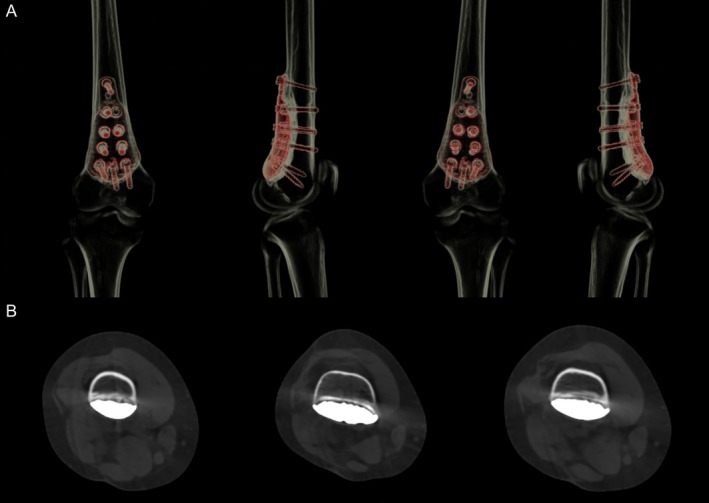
Postoperative radiographic and 3D CT evaluation of a customized 3DPSP. (A) 3D volume‐rendered CT reconstruction images of the distal femur demonstrating four different anatomical perspectives: posterior, lateral, anterior, and medial views (from left to right). The prosthesis and bicortical fixation screws are highlighted in red to facilitate visualization of their precise anatomical fit and screw positioning relative to the host bone. (B) Representative axial CT images across three different sequential levels (from left to right): proximal, midpoint, and distal. These cross‐sectional views show the interaction between the porous implant and the femoral cortex at different points along the femoral shaft.
